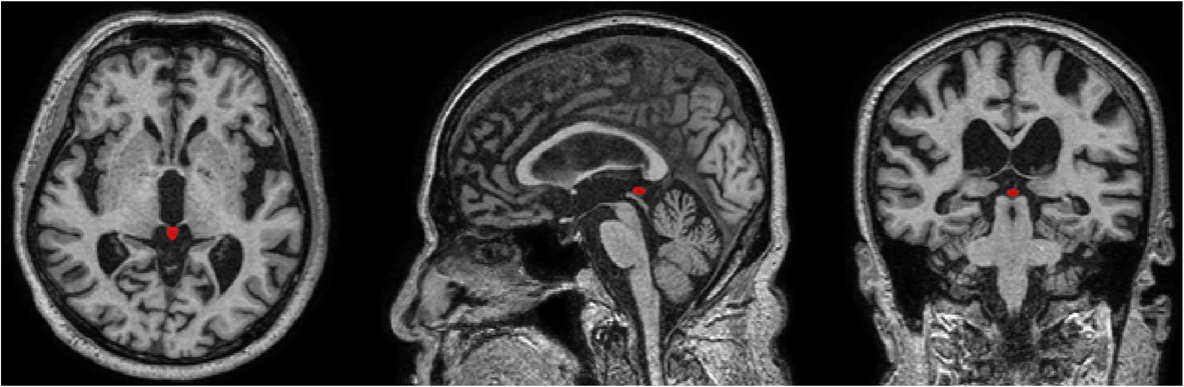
Assessment of pineal gland volume on 3D T1-weighted brain magnetic resonance images at 1.0 × 1.0 × 1.0 mm^3^. The pineal gland was manually segmented from surrounding cerebrospinal fluid space
